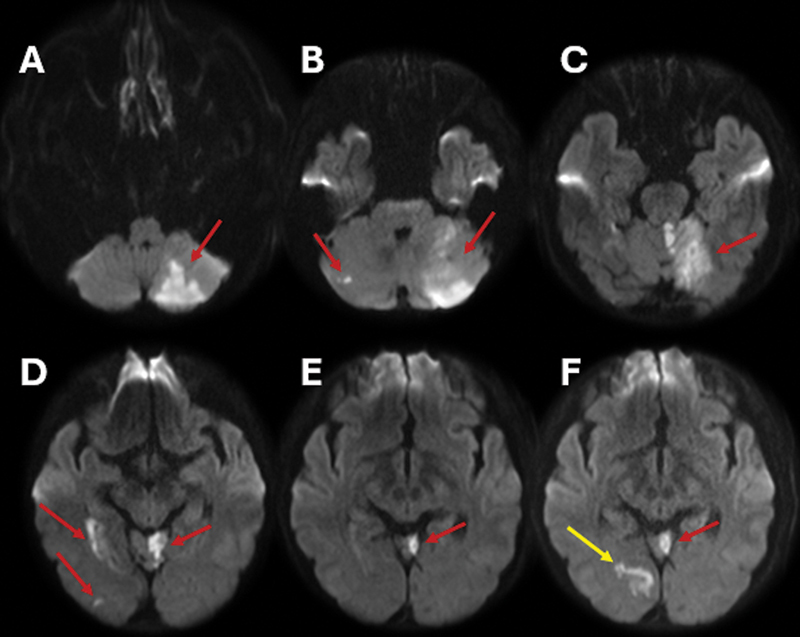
Magnetic resonance imaging (MRI) scan of the brain, axial diffusion sequence. (
